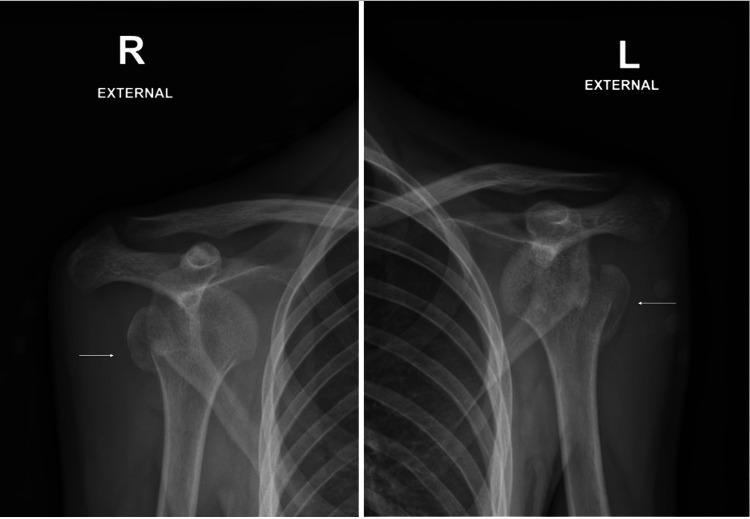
Bilateral shoulder x-rays showing anterior shoulder dislocation with proximal humerus fracture
